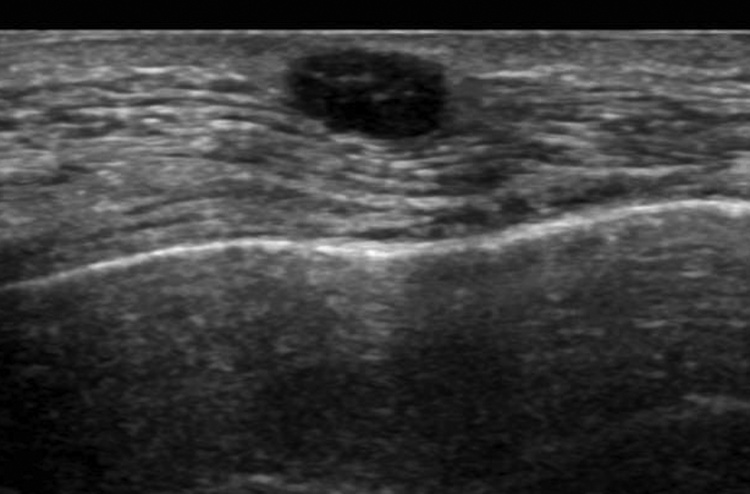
Ultrasonography showing a well-defined 8-mm diameter low-echo mass under the skin.
